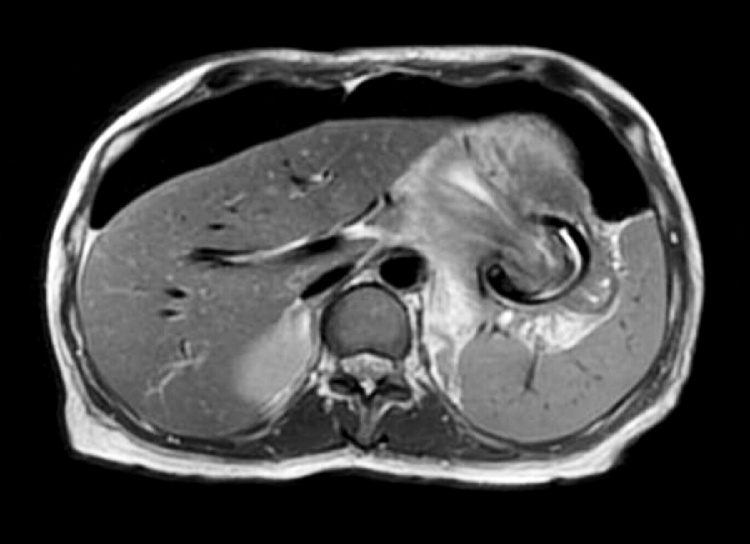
A subsequent Multi Sequential Multiplanar (MRI) of abdomen confirmed gastric band migrated inferiorly causing dilating of stomach funds with small erosions.
